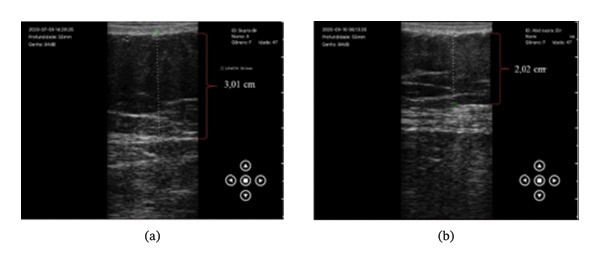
Ultrasonographic image of the abdomen of one of the participants in the G‐1 research, demonstrating the reduction of superficial adipose tissue, after the first sessions of tecar therapy.
